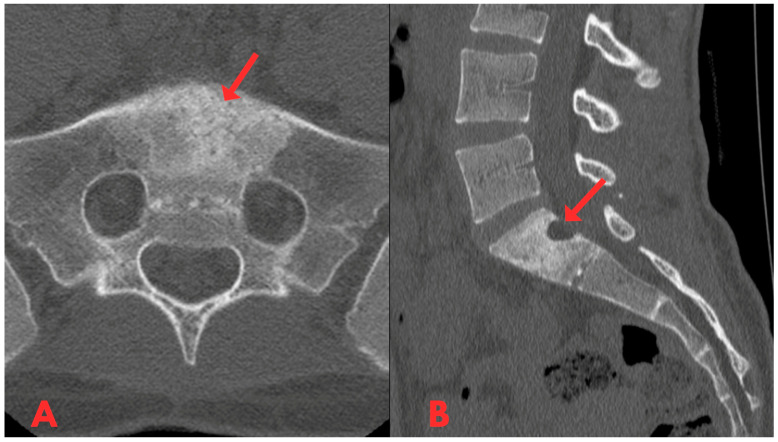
A follow-up CT examination of the lumbar spine axial and sagittal planes showing heterogeneous sclerotic changes in the body of the S1 vertebra, and compared to the previous CT and MRI scans, there were no significant changes in the dynamics of the radiological image. The posterior part of the S1 vertebral body shows a small defect after the biopsy.
